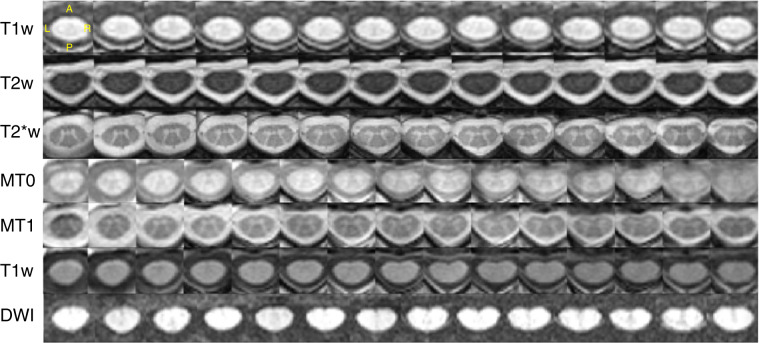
Axial views of good quality data for all sequences in the spine generic protocol across various slices (the exact coverage along the SC varies because the slice thickness varies across sequences). DWI corresponds to the mean DWI data after motion correction. The images are from different participants. T1w: *vuiisAchieva02*; T2w: *milan01*; T2*w (ME-GRE): *brnoCeitec01*; MT0, MT1, T1w (for the MTS protocol) and DWI : *barcelona04*. Axial views were automatically generated by SCT’s QC report.
